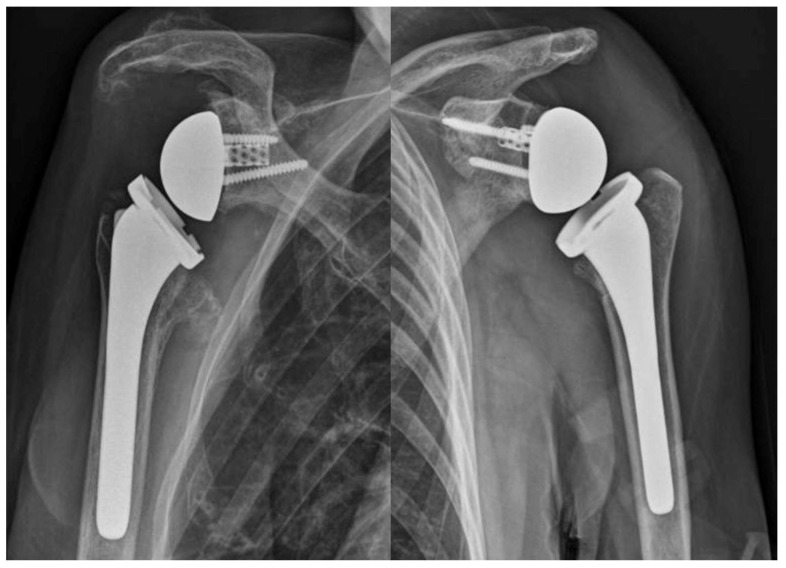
X-rays at the final follow-up.
